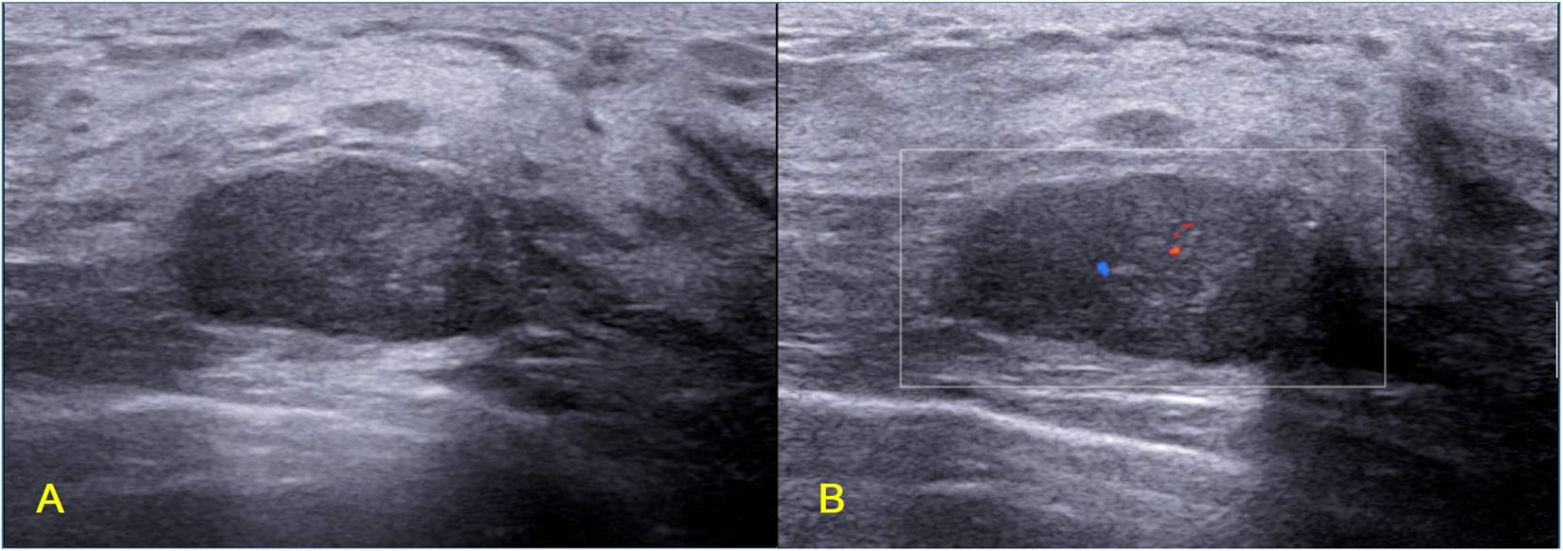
TN invasive ductal carcinoma with fibroadenoma-like sonographic features in a 25-year-old female patient (BI-RADS: 3, grade III, Ki67 90%). (A) B mode ultrasound shows a mass with paralleled orientation, regular shape, circumscribed margin and posterior acoustic enhancement; (B) Color Doppler ultrasound shows sparse blood flow signal.
